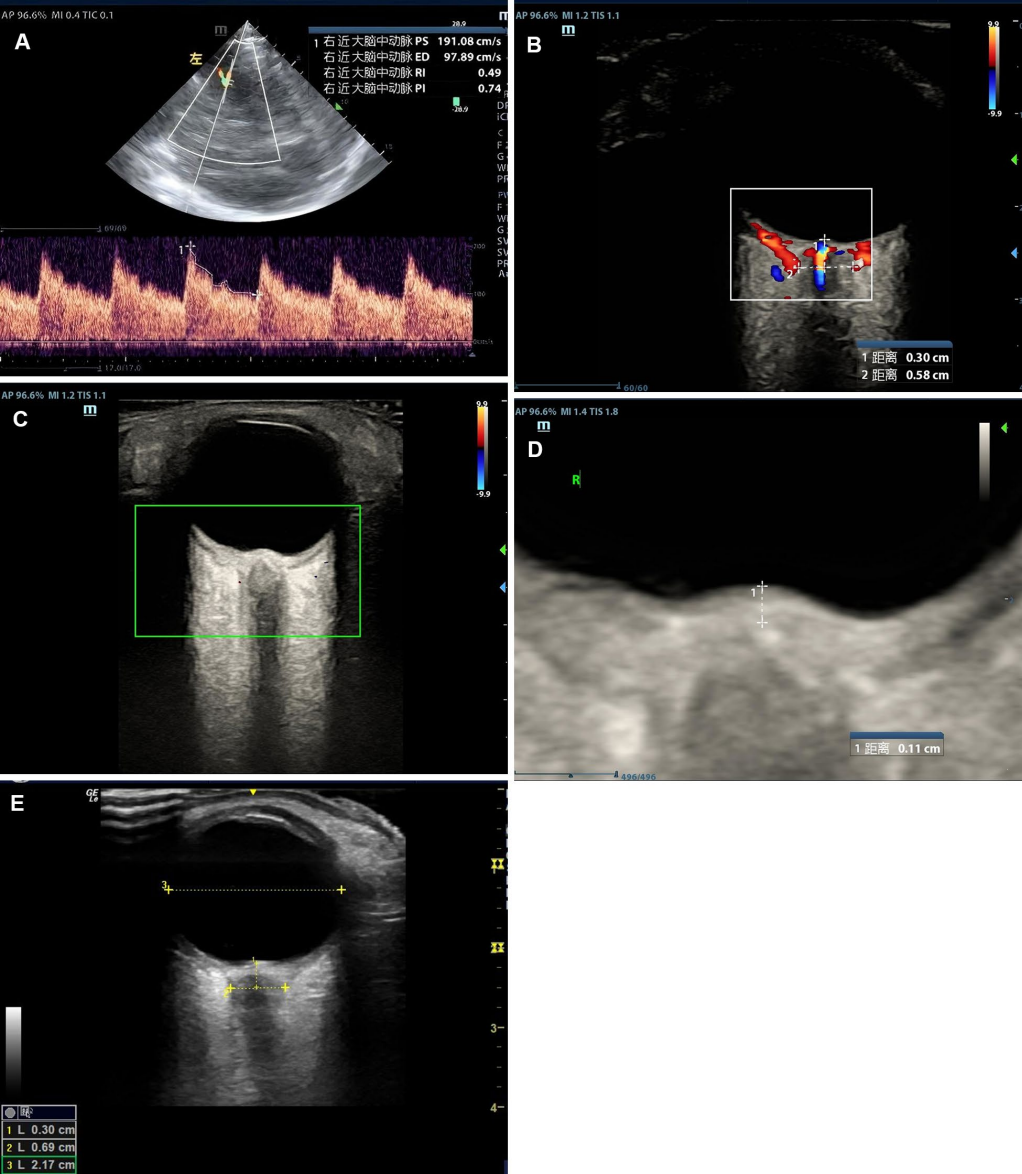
Representative ultrasound images from patients with different intracranial pressure (ICP) levels. (A) A patient with aneurysmal subarachnoid hemorrhage (ICP = 17 mmHg): transcranial color Doppler (TCCD) via the temporal window shows an end-diastolic flow velocity (Vd) of 97.9 cm/s, mean flow velocity (Vm) of 128.9 cm/s, resistance index (Ri) = 0.49, and pulsatility index (Pi) = 0.74. (B) A patient with ICP = 22 mmHg: orbital ultrasound reveals an optic nerve sheath diameter (ONSD) of 5.8 mm measured 3 mm behind the optic disc. (C) A patient with ICP = 45 mmHg: marked papilledema is observed, indicating elevated optic disc height (ODH). (D) Magnified view of the optic disc in (C), with ODH measured at 1.1 mm. (E) A patient with ICP = 34 mmHg: ONSD = 0.69 cm and eyeball transverse diameter = 2.17 cm, yielding an ONSD/ETD ratio of 0.32. All images were obtained with written informed consent from the patients
